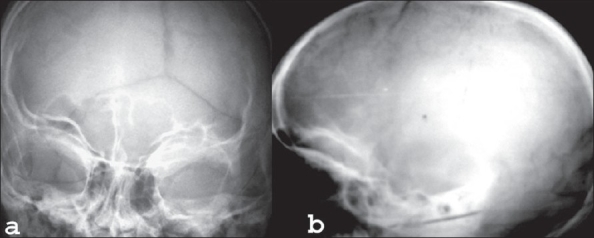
X-ray of the skull, AP (a) and Lateral (b) views showing multiple wormian bones, diastasis of the sagittal suture (a) and prominent vascular markings (b)
